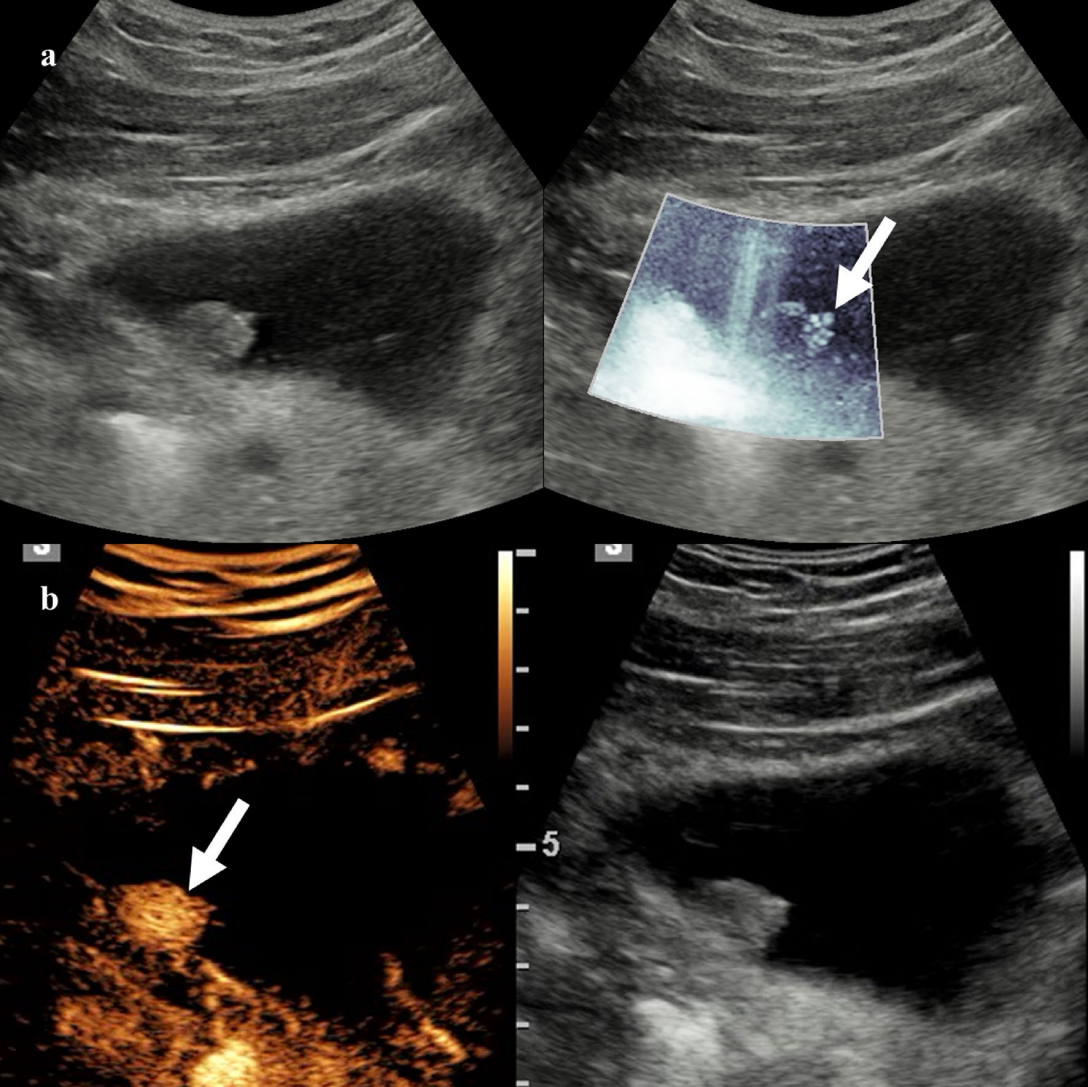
64-year-old man with bladder cancer. Ultrasound examination with microvascular flow imaging (a) shows a 2.3 cm lesion of the bladder wall with intralesional vascularization (arrow). The lesion demonstrates strong enhancement on contrast-enhanced ultrasound at 50 s after contrast injection (b, arrow)
